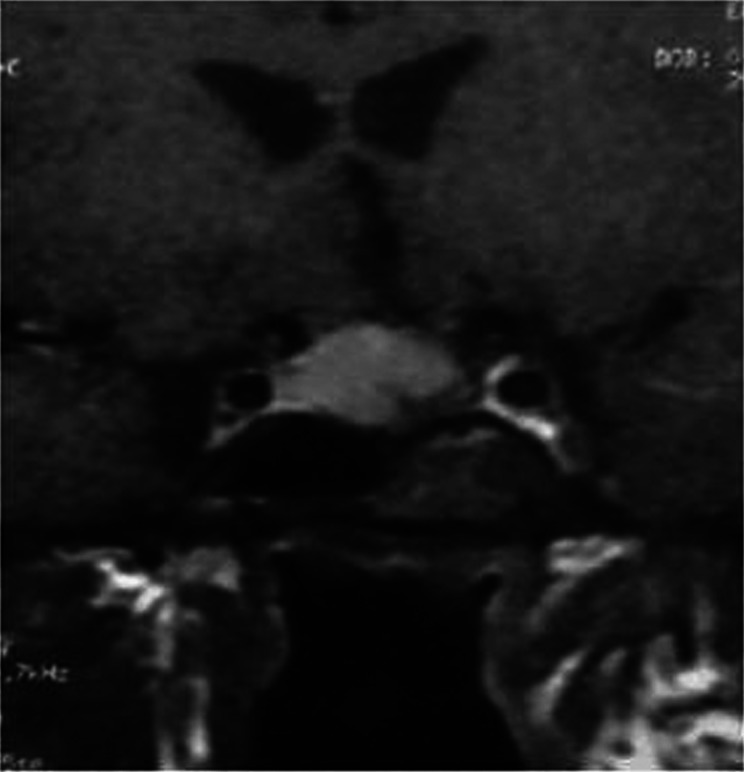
MRI of the sellar region at 4th month after the first operation.
